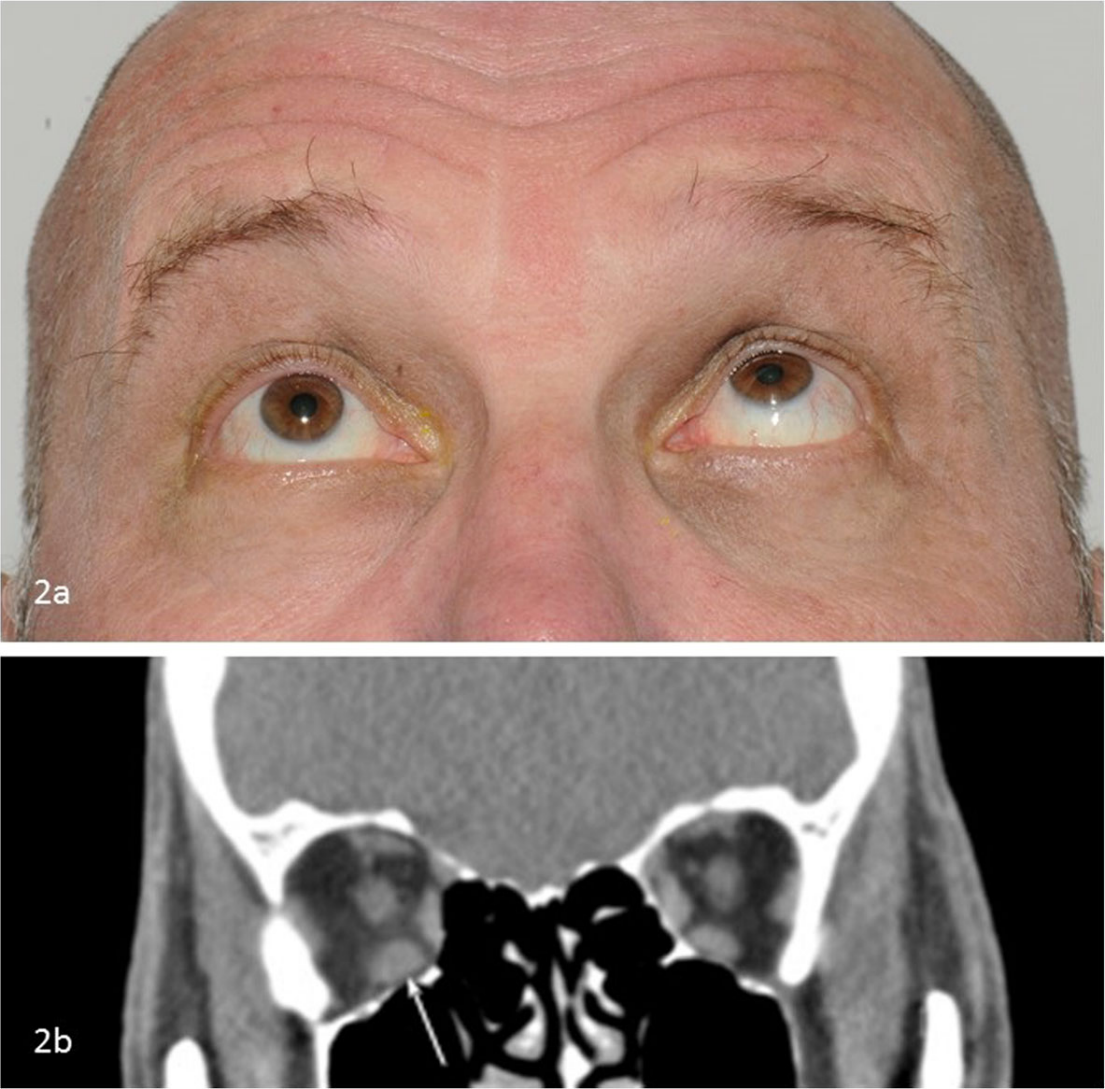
a Orthoptic evaluation now shows impaired elevation and abduction of the right eye. b CT image (coronal reconstruction) which shows enlargement of the right inferior rectus muscle with inflammatory fat infiltration surrounding the muscle
